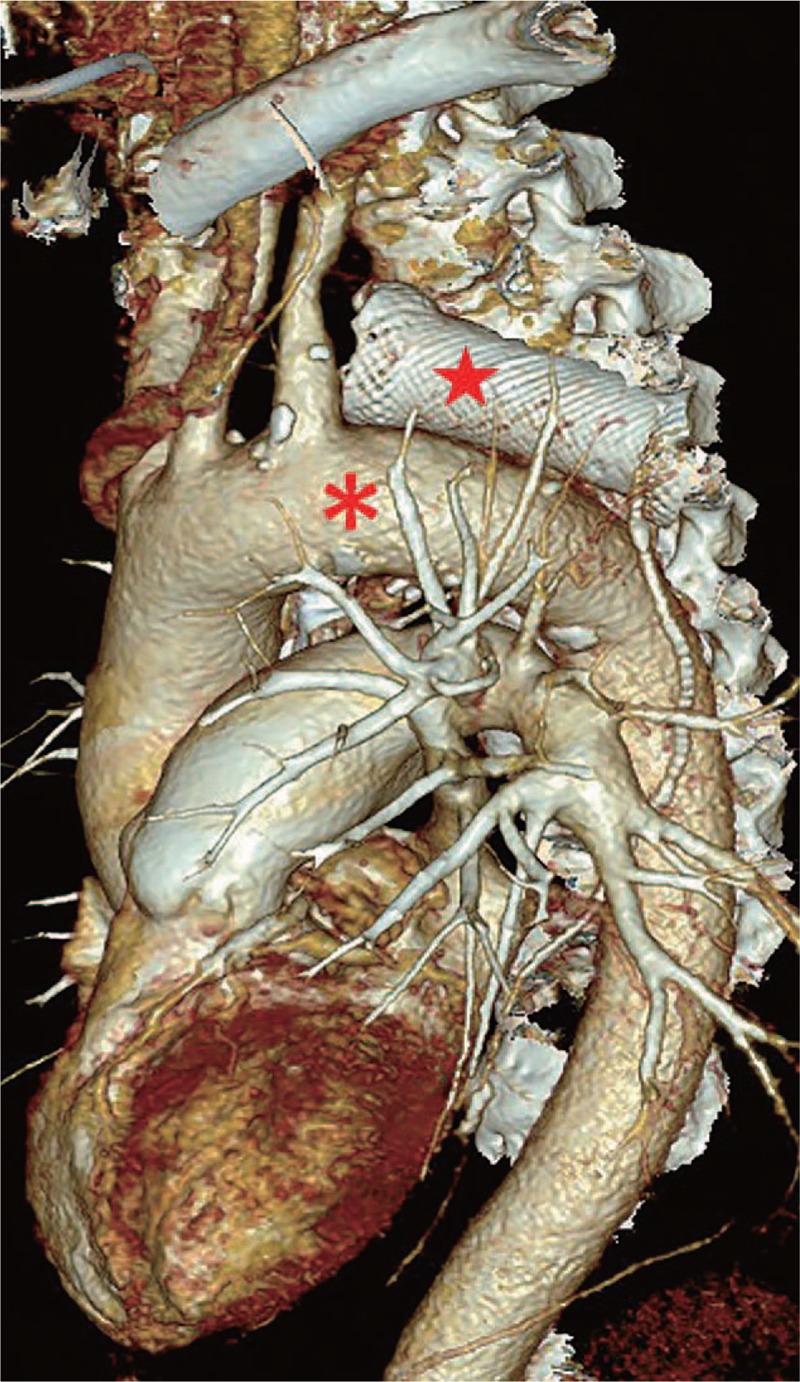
The reconstructed computed tomographic image revealed that the esophageal stent (red star) is placed close above the aortic arch (red asterisk).
